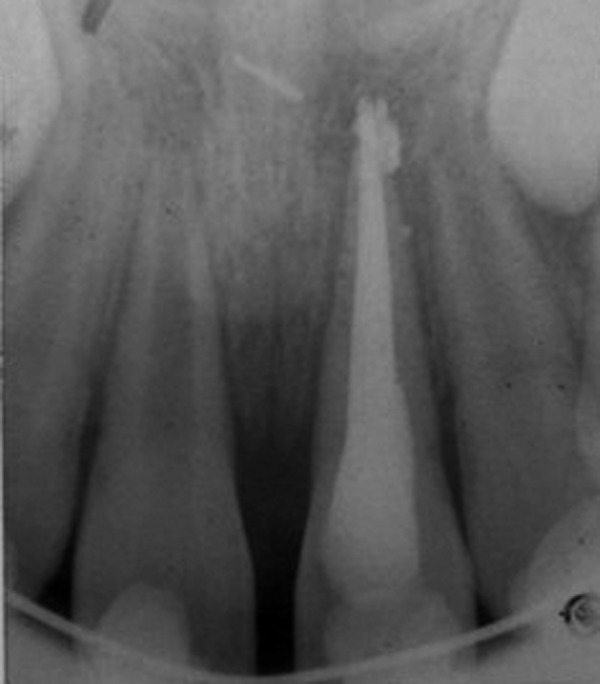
Postoperative intraoral periapical radiograph (case 4)
